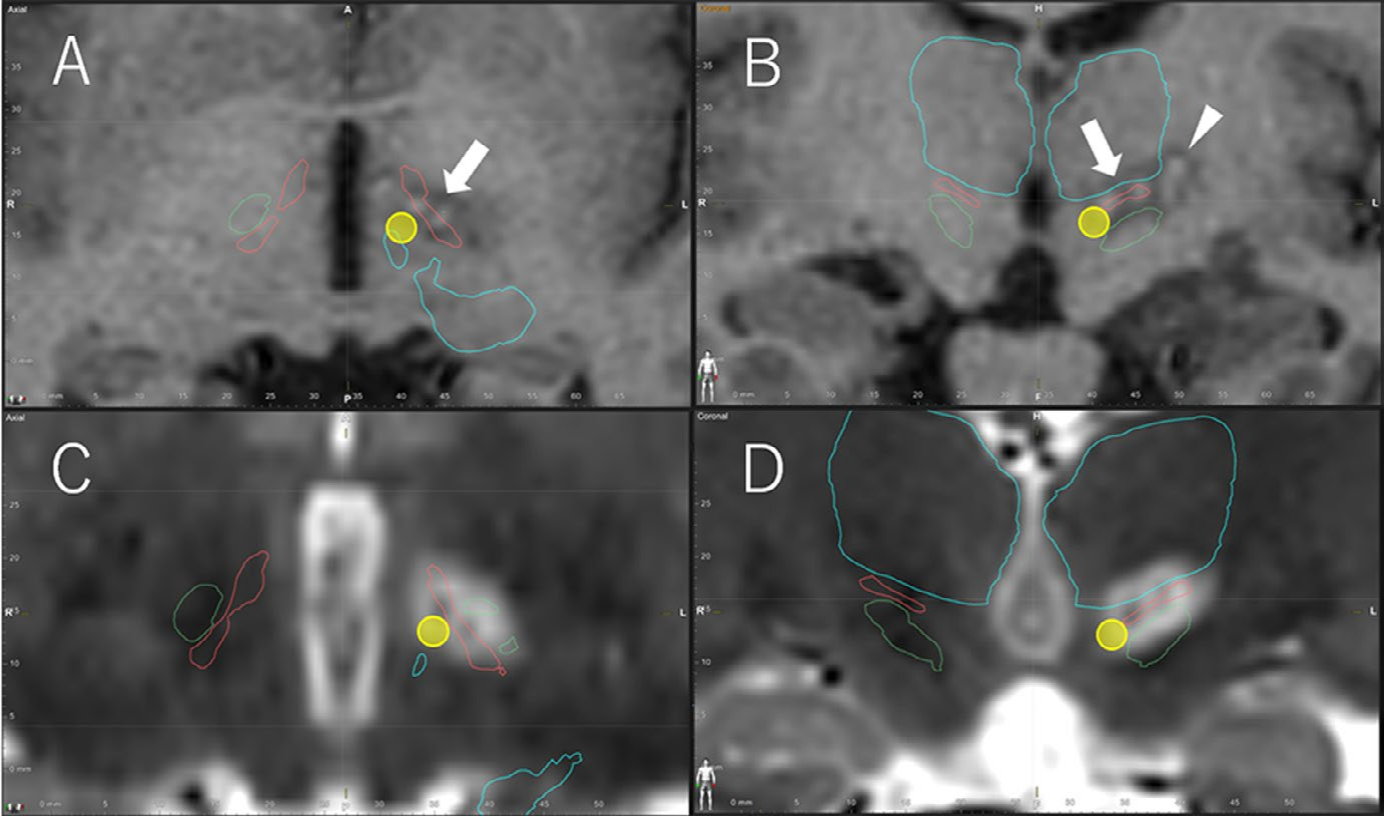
Postoperative MRI images after pallidothalamic tractotomy (Forel‐H‐tomy) in cases 1 (A, B) and 2 (C, D). A and B, Postoperative T1‐weighted axial (A) and coronal (B) MRI images in case 1 showing pallidothalamic lesions (arrow) located between the thalamus and subthalamic nucleus. A ventro‐oral lesion (arrowhead) is located lateral to the pallidothalamic lesions. C and D, Postoperative T2 weighted axial (C) and coronal (D) MRI images in case 2 showing pallidothalamic lesions located between the thalamus and subthalamic nucleus. Yellow circles represent Jinnai's Forel‐H‐tomy locations. The Brainlab Elements Anatomical Mapping Tool was used to delineate anatomical structures surrounding Forel's field H. blue: thalamus; green: subthalamic nucleus; red: zona incerta. MRI, magnetic resonance imaging
